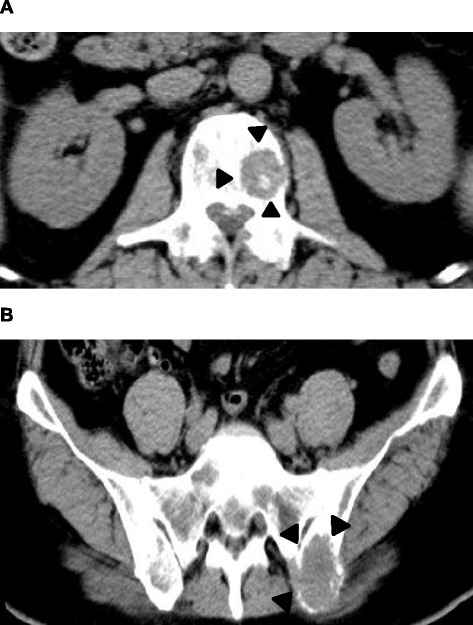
Non-contrast abdominal computed tomography (CT) images. a: Multiple bone masses; b: Multiple bone masses (*black arrowheads*) from the abdominal area
